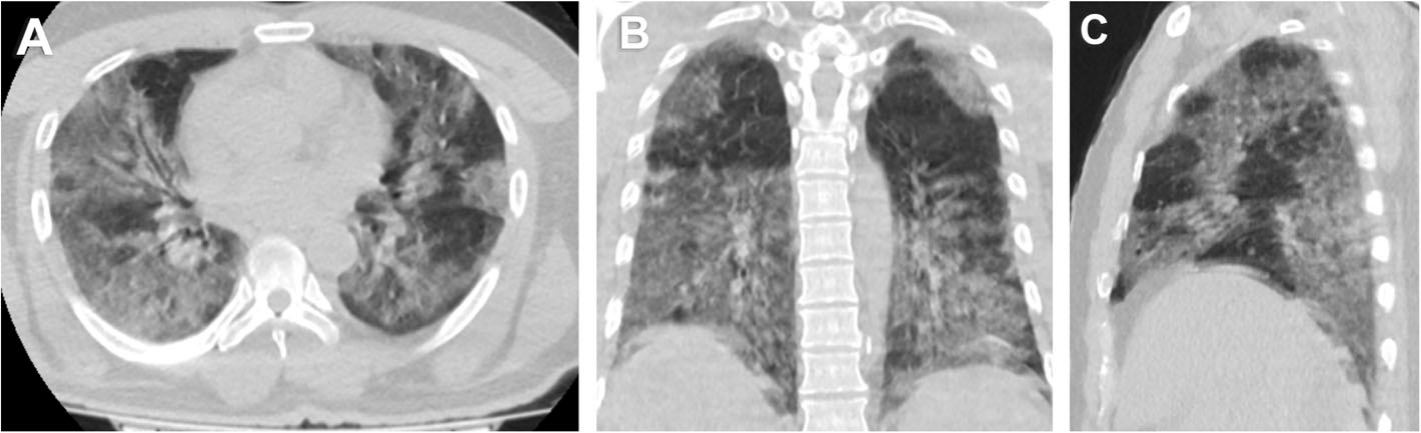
A 52-year-old male patient. A axial, B coronal, and C sagittal chest CT images show bilateral diffuse ground-glass opacities and consolidation with extensive pulmonary involvement (> 75%) corresponds to current chest CT score 20 and simple chest CT score 5 (extensive affection). The patient died on the sixth day
